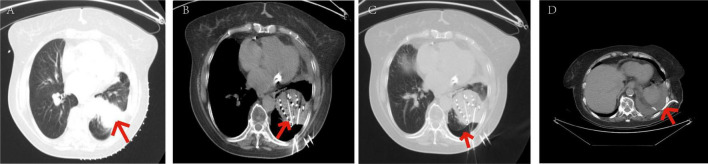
Symptoms relieved in patients with moderate pneumothorax through closed chest tube drainage. The patient, an elderly male, diagnosed with adenocarcinoma of the left lower lobe of the lung. Under our department’s care, underwent percutaneous ^125^I particle implantation close-range radiotherapy. (Indicated by the red arrow) (a) preoperative CT lung window showing a lesion measuring 7.3 × 4.9 cm. (b) Intraoperative mediastinal window showing the implantation of 109 particles in the lesion of the left lower lobe. (c) Intraoperative CT lung window showing the formation of moderate pneumothorax. (d) Postoperative closed chest tube drainage placement and drainage procedure, with approximately 1000 ml of gas drained over 3 days, leading to significant improvement in symptoms. On the 4th day, the closed chest tube drainage was removed, with a small amount of pleural effusion in the left lower lobe.
